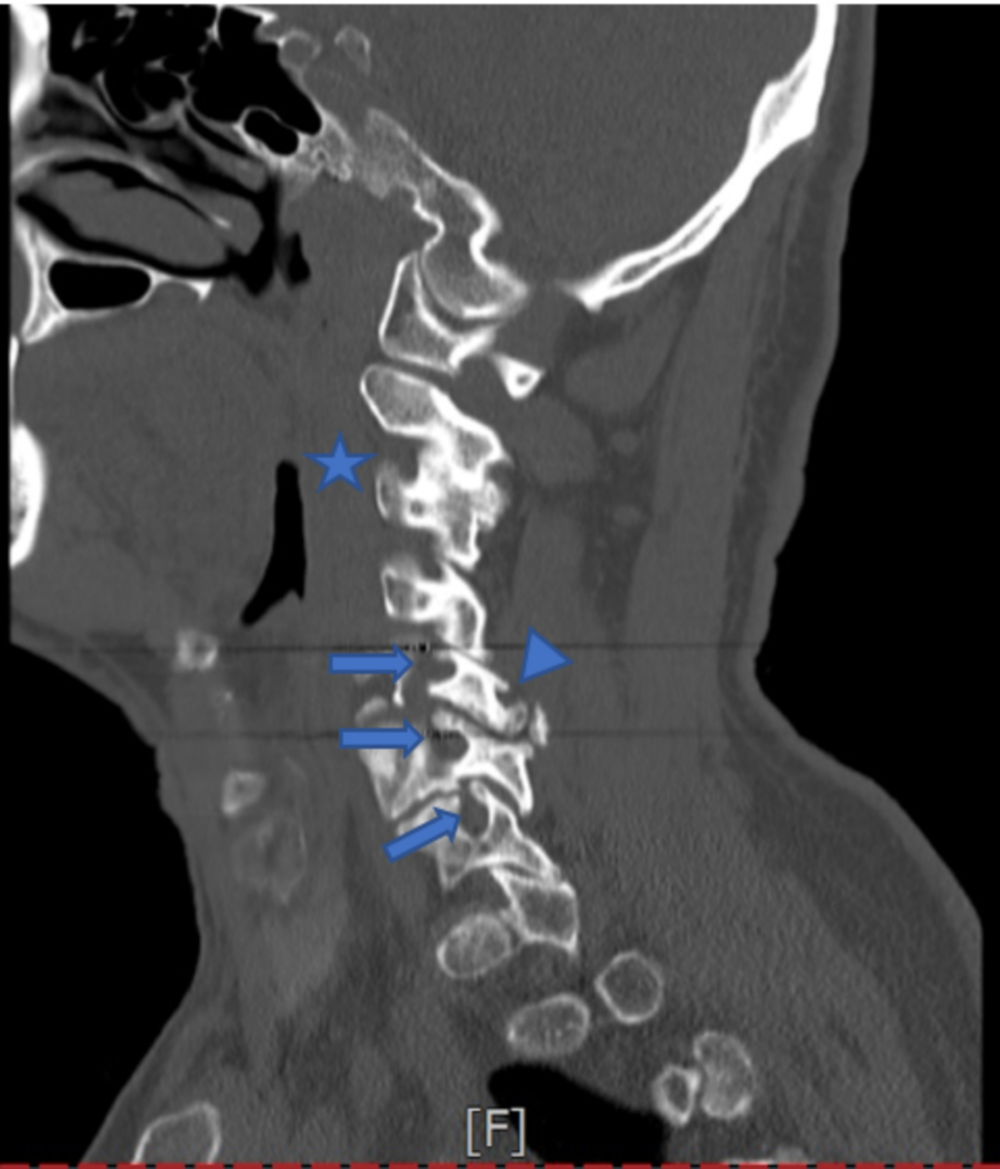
CT of the cervical spine Left posterior fusion of C2-C3 (star). Moderate to severe canal stenosis of C4-C5 and C5-C6 (arrows). At C5-C6, there is grade 1 anterolisthesis with uncovering of the disc and broad-based disc osteophyte complex (triangle). At C6-C7, there is broad-based osteophyte complex and bilateral uncovertebral. CT: computed tomography
